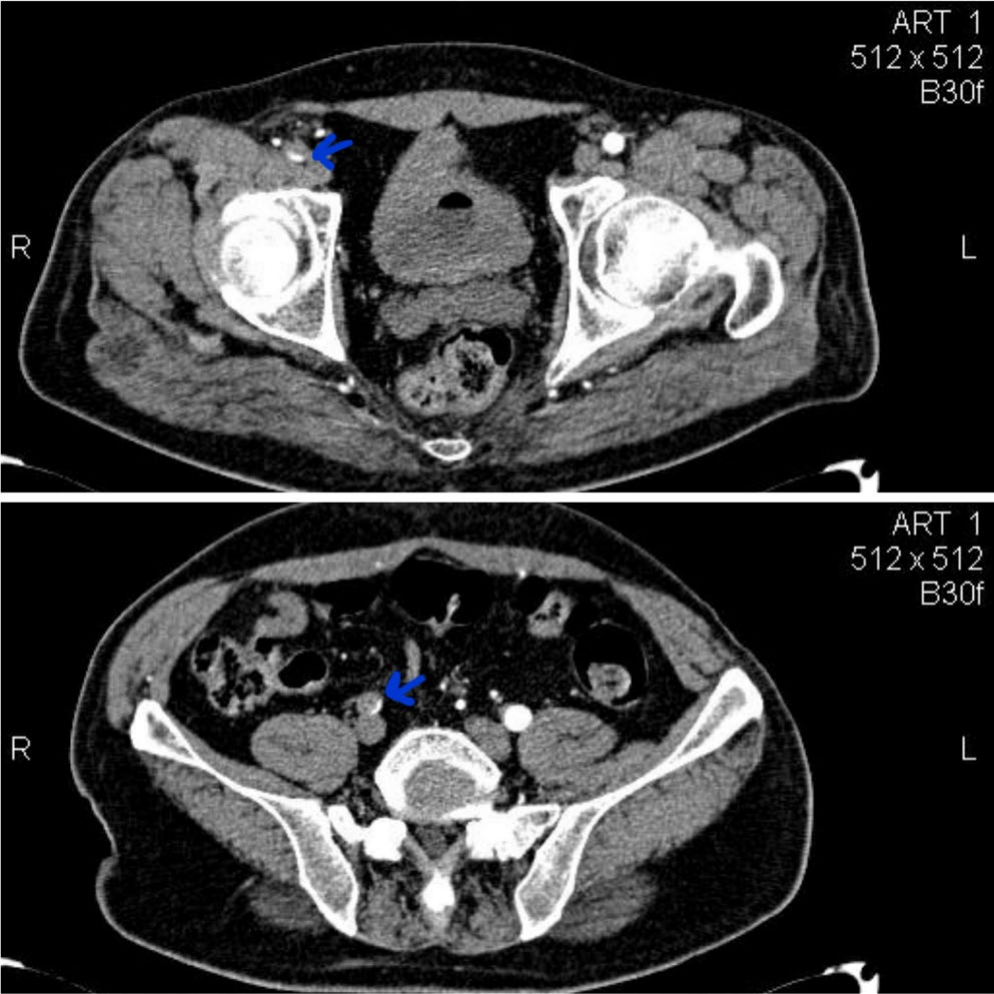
CT angiography showed thrombotic occlusion at the right common iliac artery, right external iliac artery, and common femoral artery (blue arrows)
